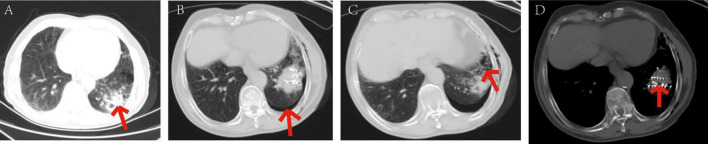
Patients with mild pneumothorax did not receive any specific treatment. The patient, an elderly female, diagnosed with adenocarcinoma of the left lower lobe of the lung. Under our department’s care, underwent CT-guided percutaneous ^125^I particle implantation close-range radiotherapy. (Indicated by the red arrow) (a) preoperative CT lung window showing a lesion measuring 5.6 × 6.2 cm. (b) Immediate postoperative CT reexamination showing mild pneumothorax formation in the lung window. (c) Intraoperative CT lung window showing pulmonary emphysema adjacent to the lesion in the left lower lobe and cavities visible within the lesion. (d) Intraoperative mediastinal window showing the implantation of 91 particles in the lesion of the left lower lobe. The patient’s mild pneumothorax was self-absorbed, and on reexamination 2 days later, the pneumothorax had completely disappeared.
